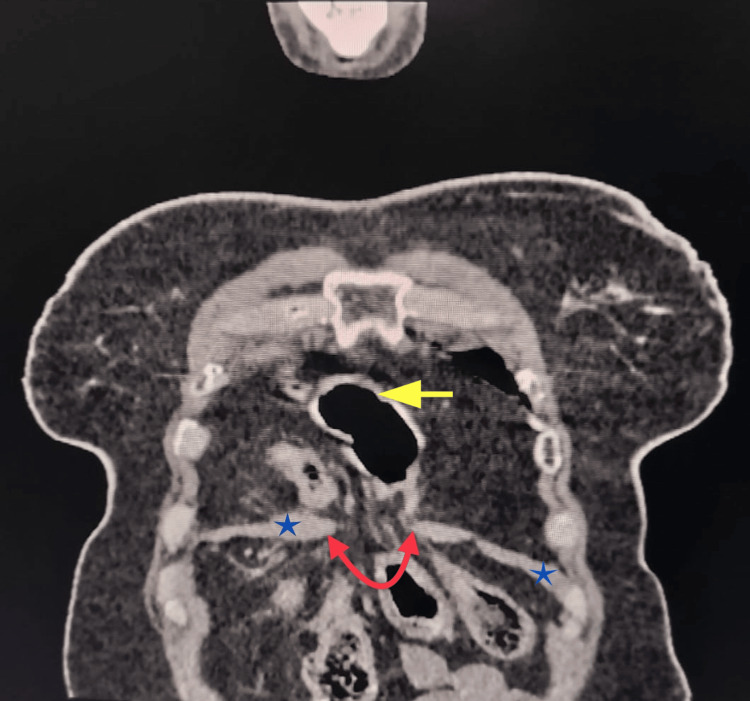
Coronal view of CT showing diaphragmatic defect (red arrow) through which the abdominal content (large bowel, marked with a yellow arrow) has herniated into the thoracic cavity, while the diaphragm is marked with blue asterisks.
